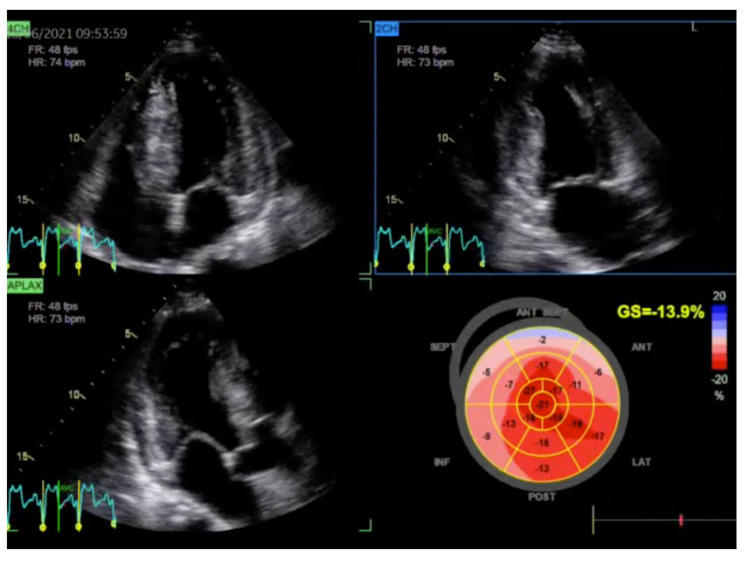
Longitudinal left ventricular strain assessment in patient with hypertrophic cardiomyopathy. Two-dimensional transthoracic echocardiogram. Moderately reduced strain (−13.9%).
